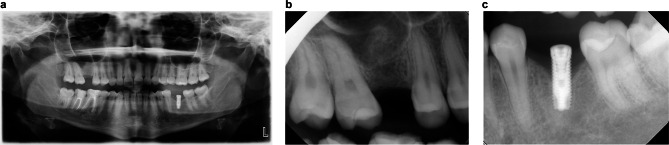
radiographs taken at the first dental visit. a Panoramic radiograph shows all teeth are present except teeth #16 and 36 and an implant at the area of tooth #36. b Bone graft at the right maxillary sinus is evident, indicative of indirect sinus lift procedure on previous implant placement attempts in area of tooth #16. c Implant at the area of tooth #36. Crestal bone loss is seen with thin radiolucent line around the implant body. Implant is migrated to a more coronal position as a result of loss of osseointegration
